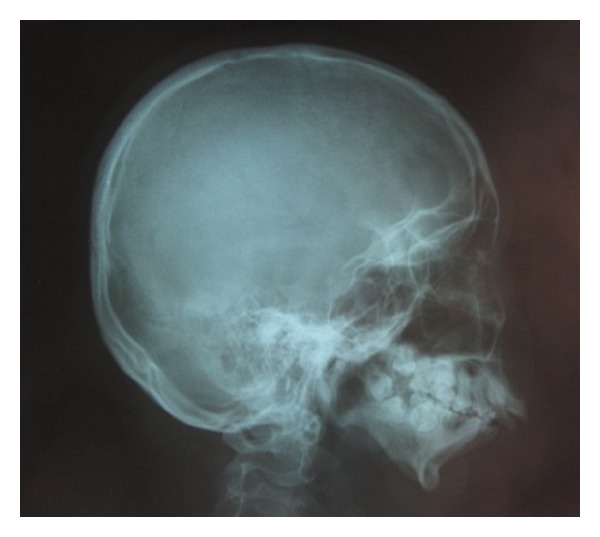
Lateral skull. Radiograph shows severe retruded mandible.
